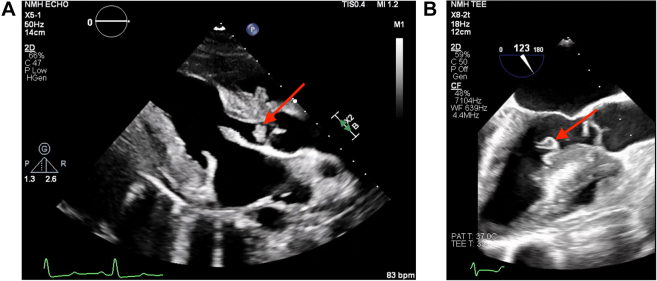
Echocardiographic Visualization of Intracardiac Lesions (A) Transthoracic echocardiography showing round mobile echodensity attached to the aortic valve (arrow). (B) Transesophageal echocardiography showing large echodensity attached to the basal anteroseptal region of the left ventricle (arrow).
